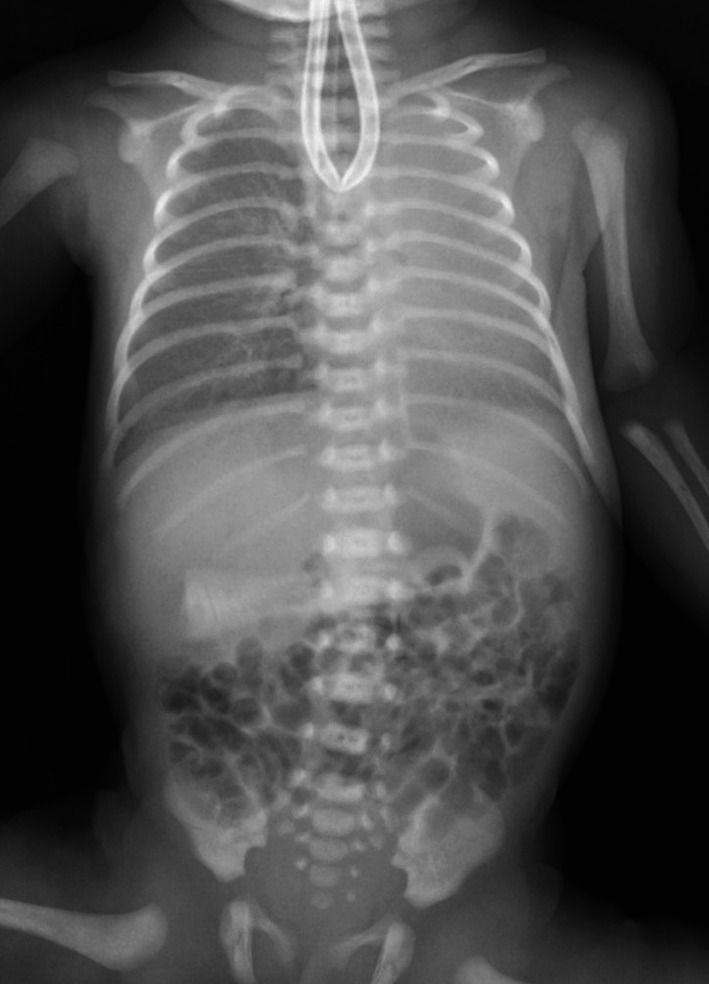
Chest radiography taken after birth showing folding of the nasogastric tube in the upper pouch and hypoplasia of the left lung
